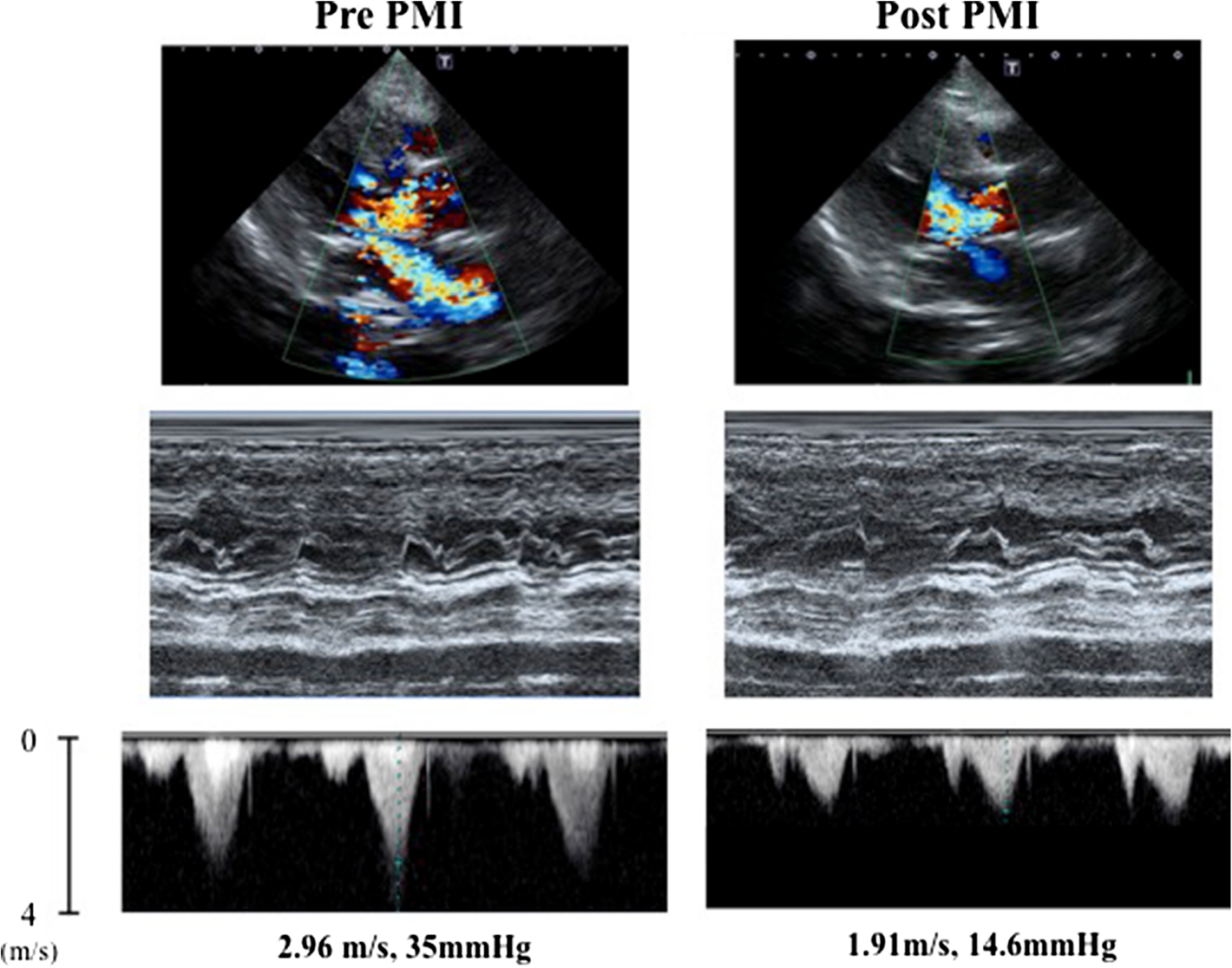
Transthoracic echocardiography demonstrated severe MR with SAM and LVOT obstruction (LVOT pressure gradient, 35 mmHg) pre-PMI. After PMI, transthoracic echocardiography showed significant improvements in MR and LVOT obstruction (LVOT pressure gradient, 14.6 mmHg). *LVOT* left ventricular outflow tract, *MR* mitral valve regurgitation, *SAM* systolic anterior motion
